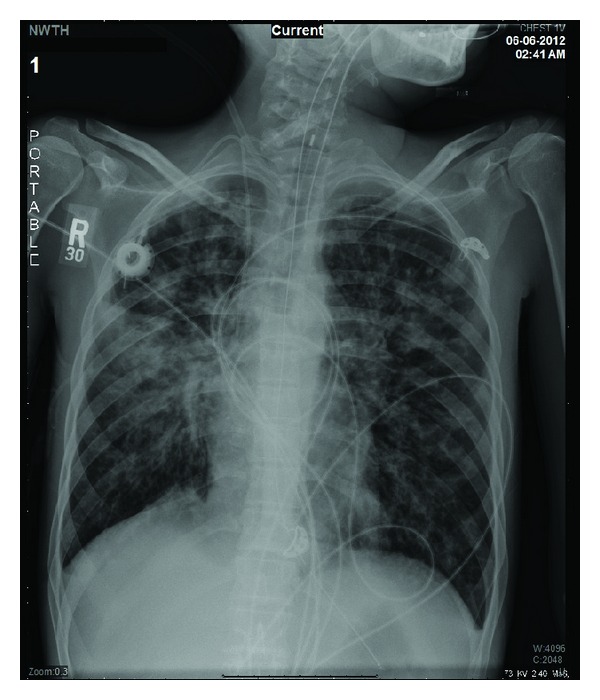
Chest X-ray on ICU admission.
